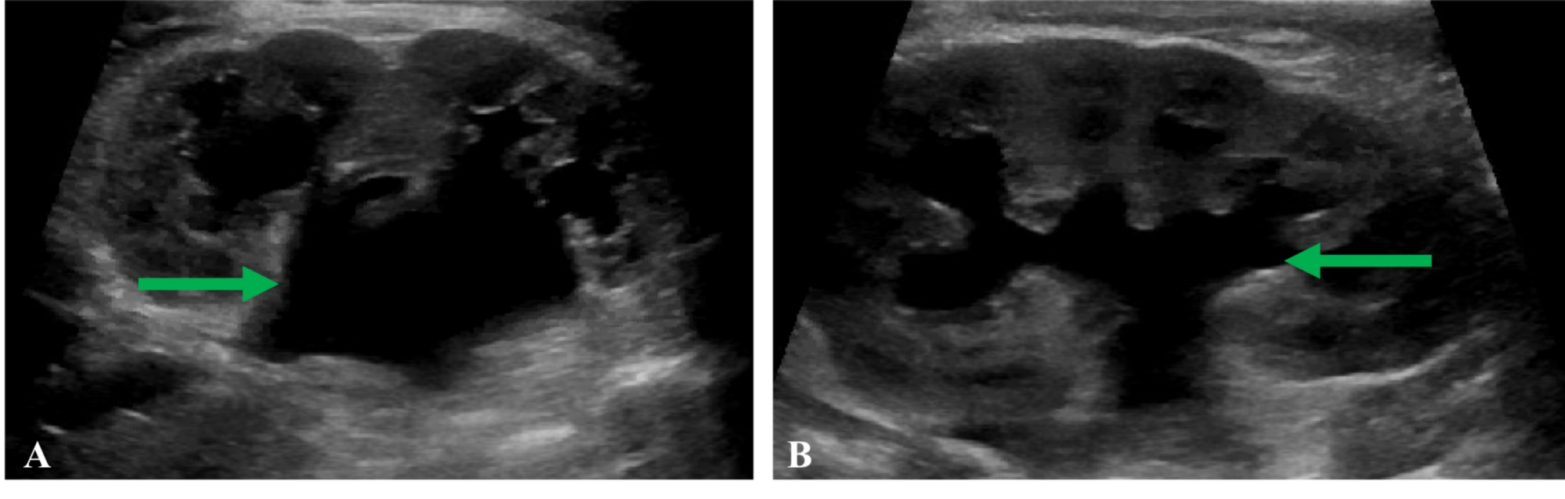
Initial renal ultrasound demonstrating bilateral moderate hydronephrosis (A) Right kidney with moderate hydronephrosis (green arrow). (B) Left kidney with moderate hydronephrosis (green arrow).
